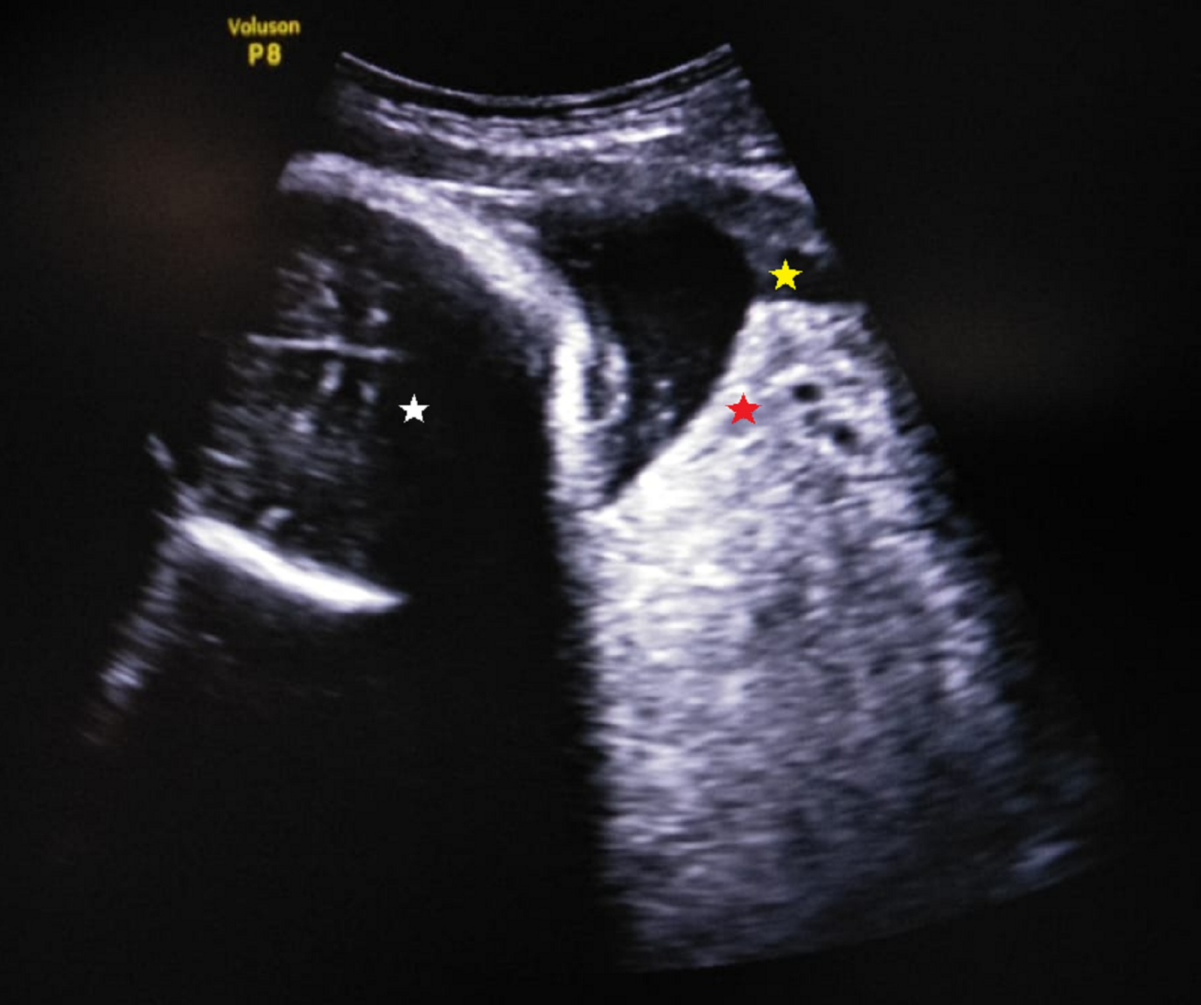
Ultrasound of pelvis. Ultrasound image showing cervical fibroid (red star) of size 13 cm x 10 cm arising from the posterior cervix and bulging into the cervical canal (yellow star). The fetal head (white star) can be seen above the fibroid mass.
